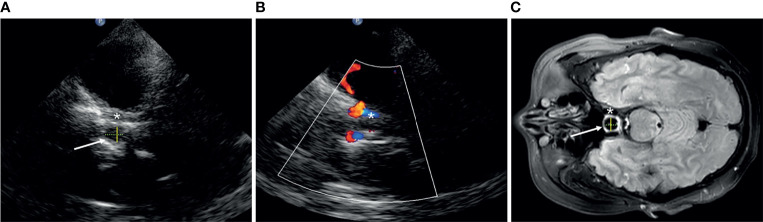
The structure of Sella turcica (arrow) in transcranial sonography (A, B) and MRI (C). Asterisk, cavernous segment of the internal carotid artery; yellow solid line, D1; green dotted line, D2.
